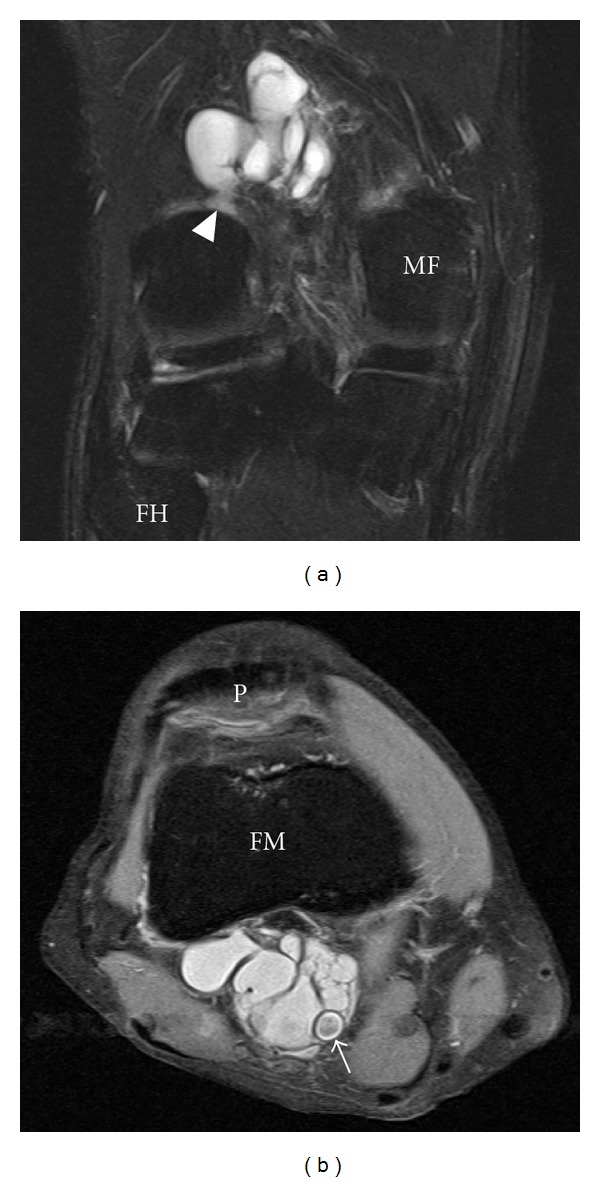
Cluster of communicating extra capsular synovial cysts at the dorsal aspect of the femoral metaphysis. (a) Coronal fat-saturated T2 and (b) axial proton density fat-saturated MR images of the right knee without effusion in a 51-year-old male floor layer. The lesion takes it origin from the intracapsular synovial recess at the dorsal cranial aspect of the lateral femoral condyle through a defect in the capsule (arrow head). The majority of these lesions detected in floor layers originated similarly but from the medial femoral condyle. FH, fibular head; MF, medial femoral condyle; FM, femoral metaphysis; P, patella; arrow, popliteal artery.
